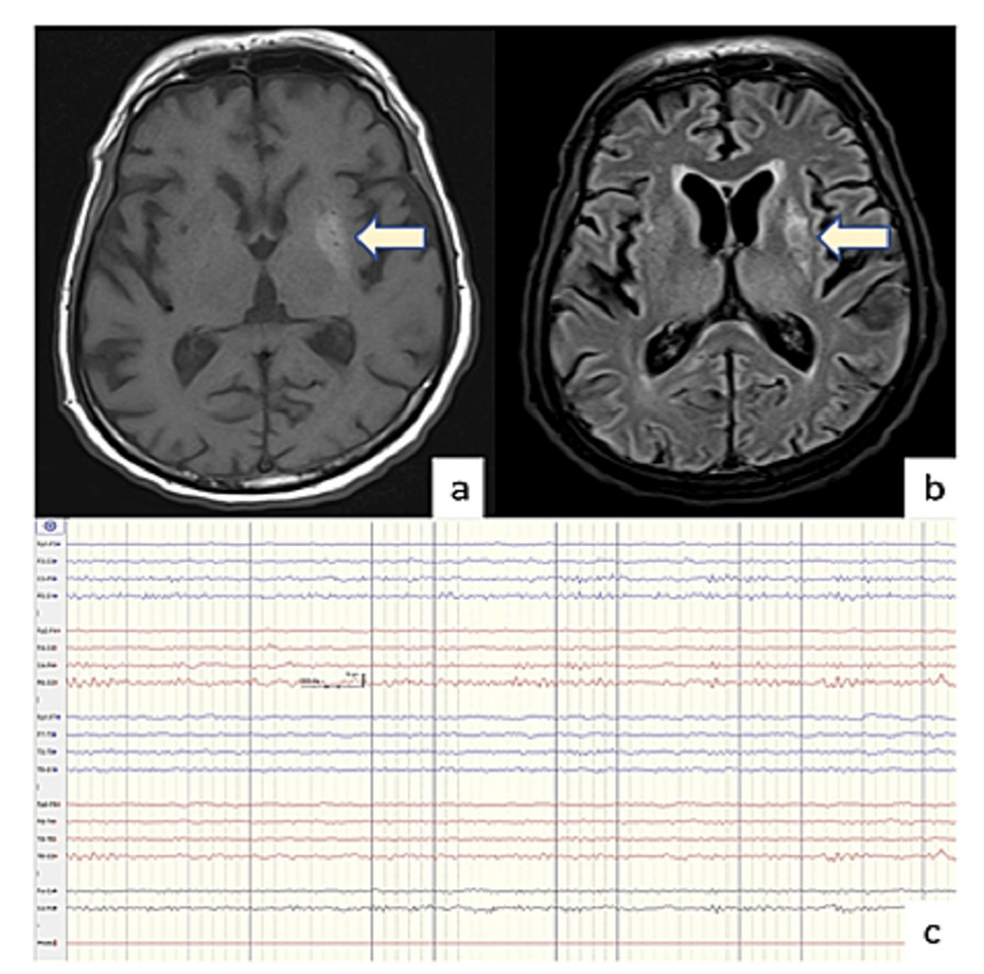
Neuroimaging (a) MRI (axial) brain of a 51-year-old female showing hyperintensity (arrow) on T1W (a) as well as onT2W (b) imaging (arrow) involving the left lentiform nucleus. (c) Normal EEG of the same patient after the resolution of abnormal limb movements.
